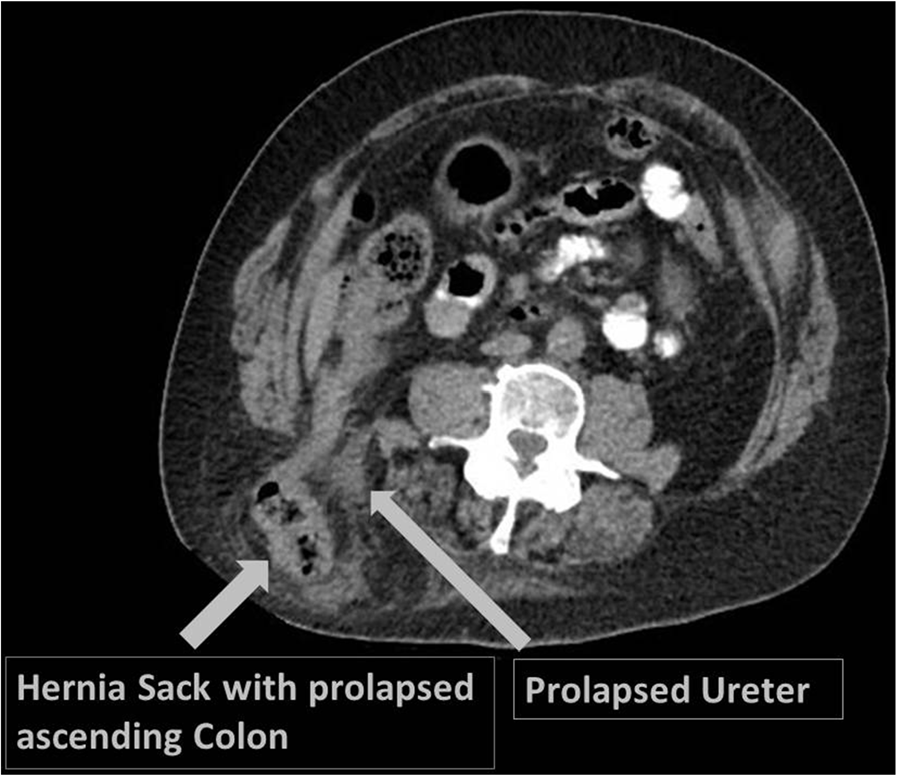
Preoperative CT revealed a large Grynfeltt hernia with prolapse of the ascending colon and the right ureter with consecutive dilatation of the renal pelvis
